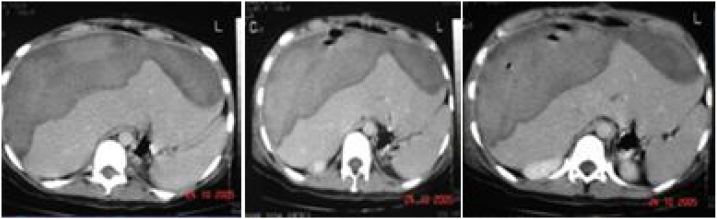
CT showing persistence of hepatic hematoma, no free liquid.
